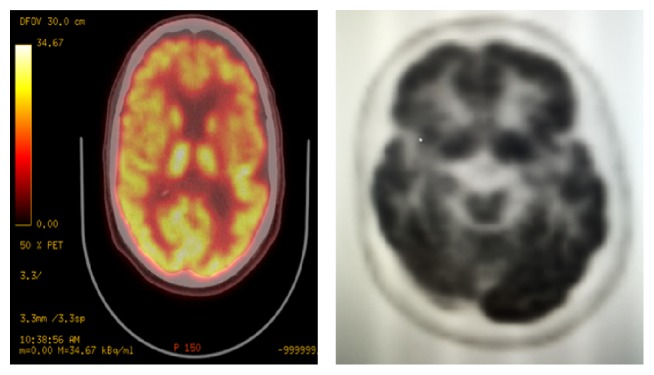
PET scan of the brain showing no hypermetabolic lesions in the brain to suggest lymphoma. Hypometabolism is seen in the right frontal operculum and left frontal vertex. There is a larger area of subtle asymmetric decreased FDG activity seen in posterior left frontal lobe and left parietal lobe.
